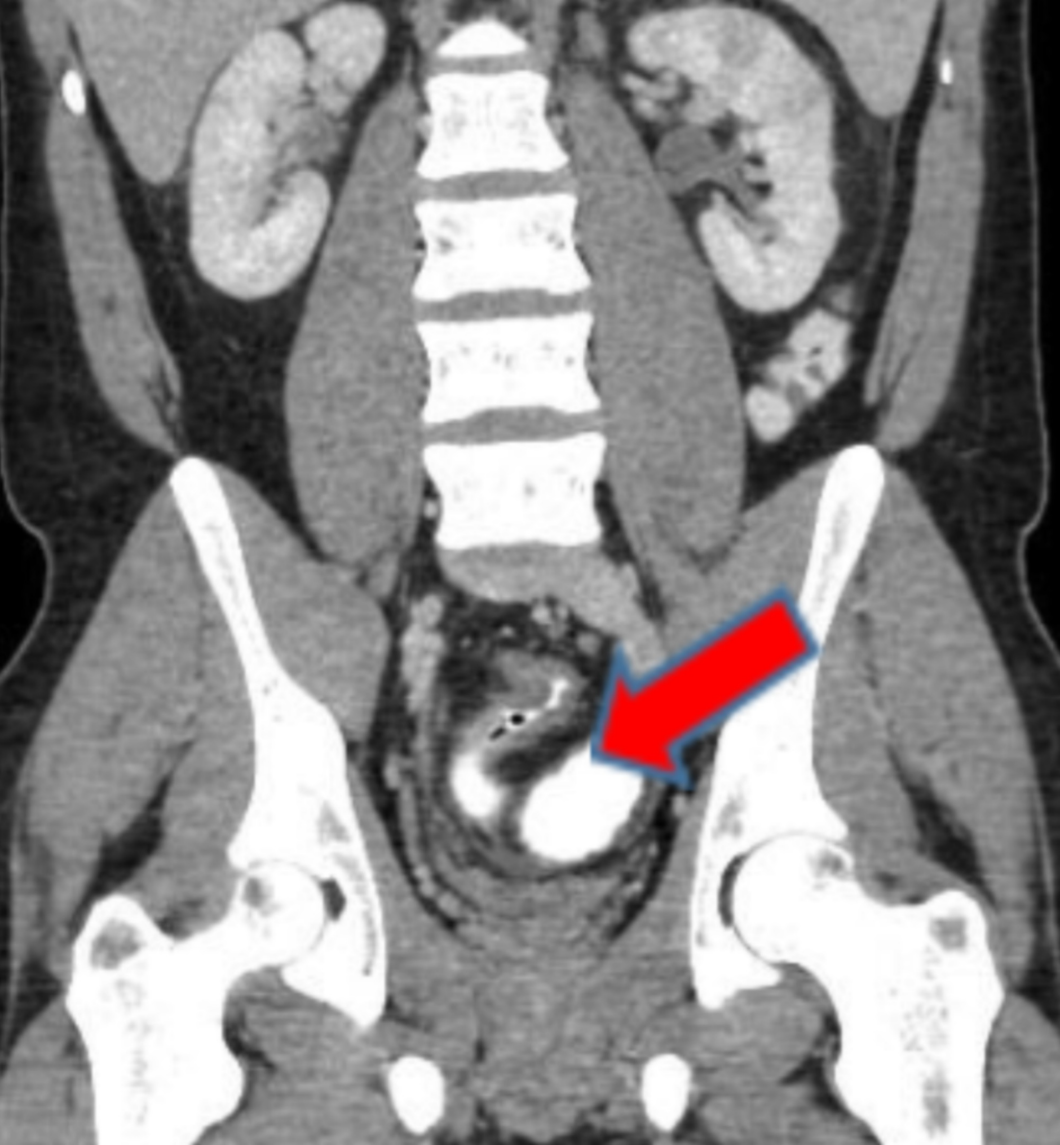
CT scan showing abnormal wall thickening of sigmoid colon indicated by the red arrow
